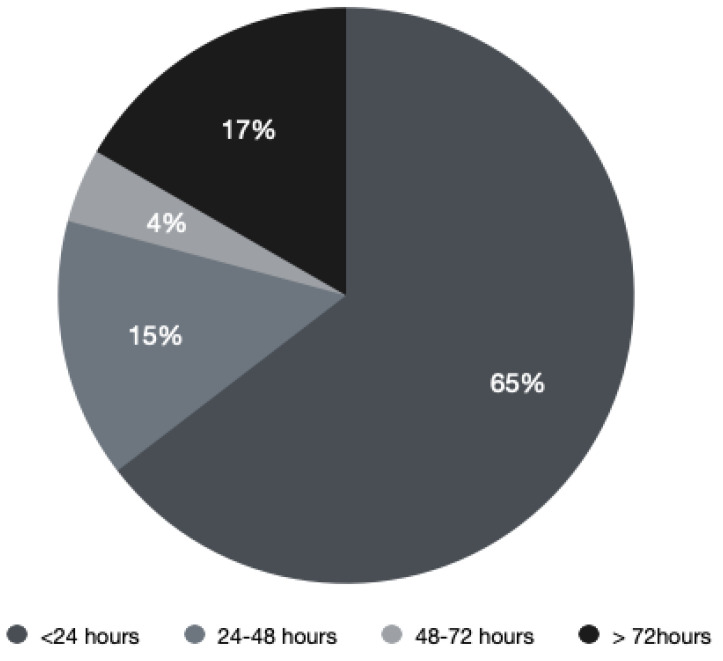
Time from admission to magnetic resonance imaging (MRI) in hours.
